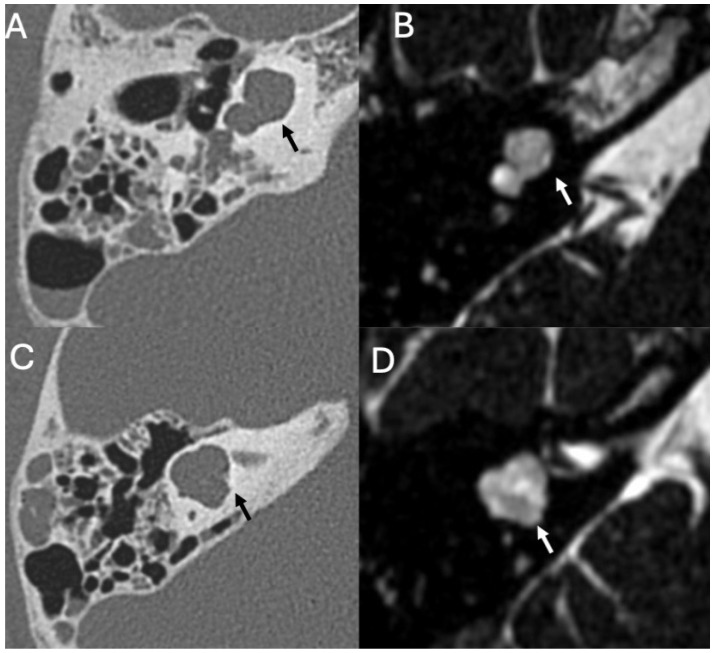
Incomplete partition type I in a 14-year-old patient with recurrent Meningitis due to *Streptococcus pneumoniae*. Axial CT (A,C) and heavily T2-weighted (B,D) images show an enlarged cochlea lacking modiolus and interscalar septa, resembling an empty cyst (arrows (A,B)). The vestibule is enlarged and dysmorphic (arrows (C,D)). Note also fluid in the mastoid (A,C).
